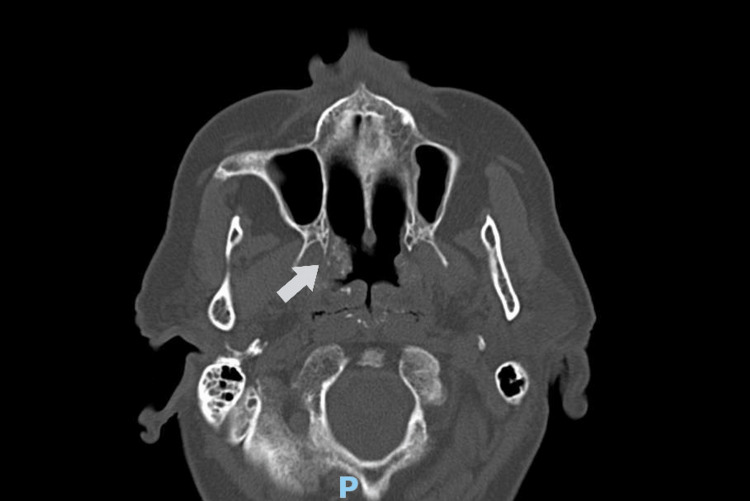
Contrast-enhanced CT scan revealing lymphoid tissue occupying the posterior end of the right nasal cavity CT: computed tomography
